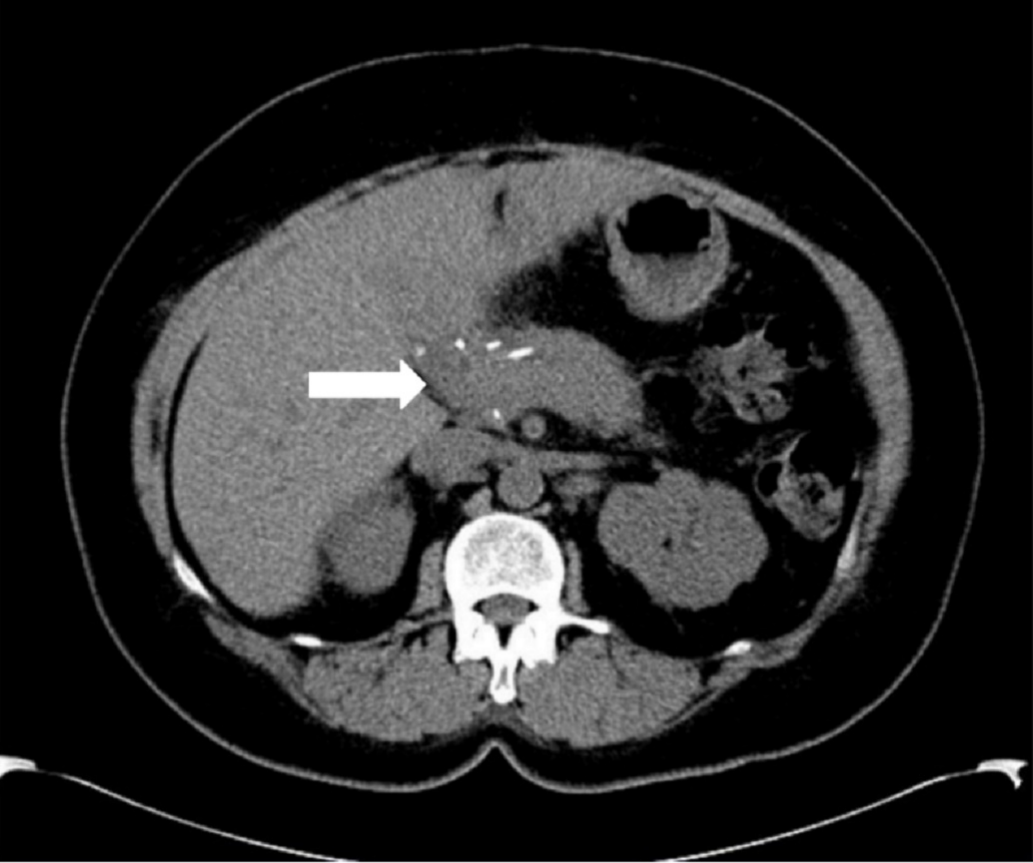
Postoperative CT scan The non-contrast enhanced CT axial image demonstrates post-Whipple changes and a hypodense lesion in the pancreatic body/tail remnant (white arrow).
